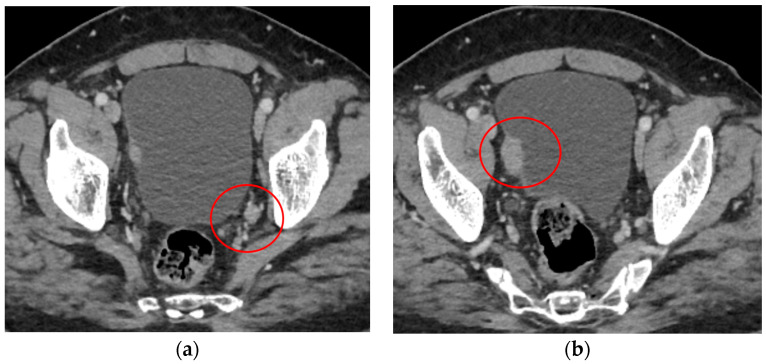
Preoperative contrast-enhanced thoraco-abdominal-pelvic CT scan. (a) Enlarged lymph node at the level of left obturator fossa (Red circle—enlarged lymph node). (b) Tumor recurrence at the level of right bladder wall (Red circle—tumor recucurrence).
